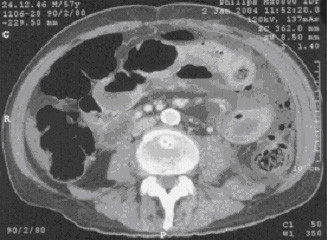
CT scan with intravenouse contrast: Free intraperitoneal air and a enlarged jejunum.
